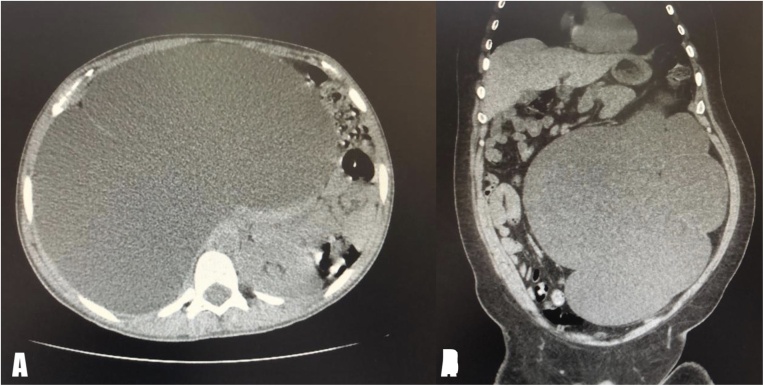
(A, B): Axial and coronal CT scan showed severe left hydronephrosis crossing the midline with thinning of renal parenchyma.
